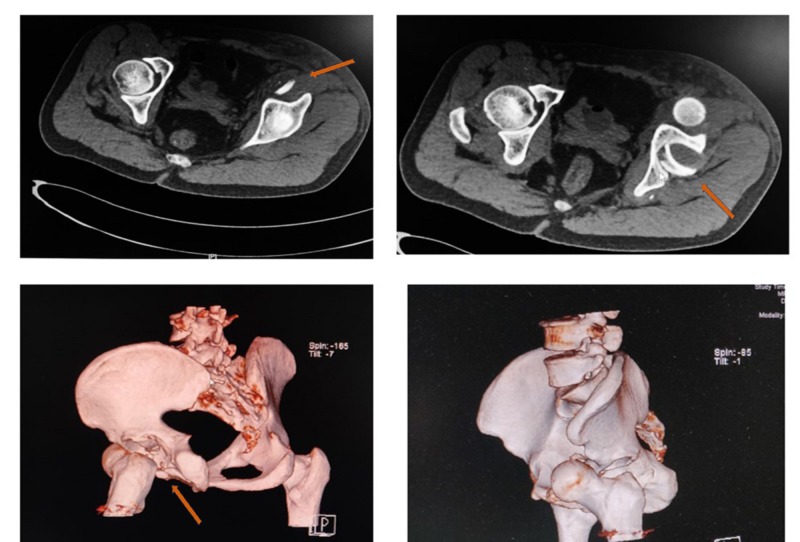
Preop CT imaging Pre-reduction coronal and 3D computed tomography (CT) scan of the left hip with the pelvis showing anterior dislocation of the left hip with the fracture of the anterior wall and the posterior wall of the acetabulum
